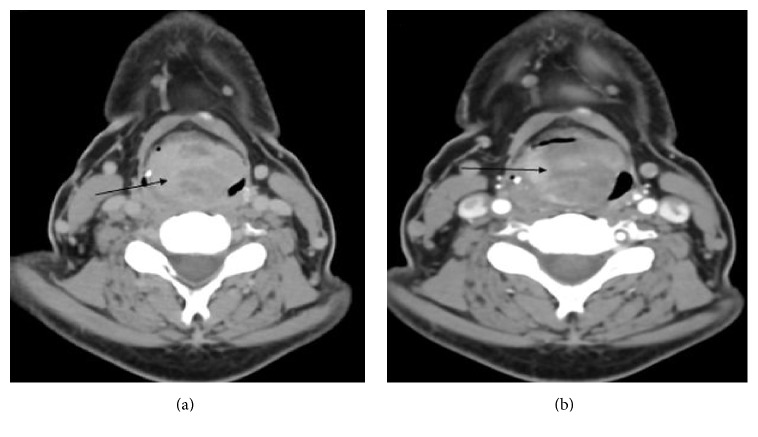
(a) Plain CT scan of the neck showing soft tissue mass at the region of epiglottis with tiny specks of calcifications in the periphery. (b) Postcontrast image showing heterogenous enhancement.
